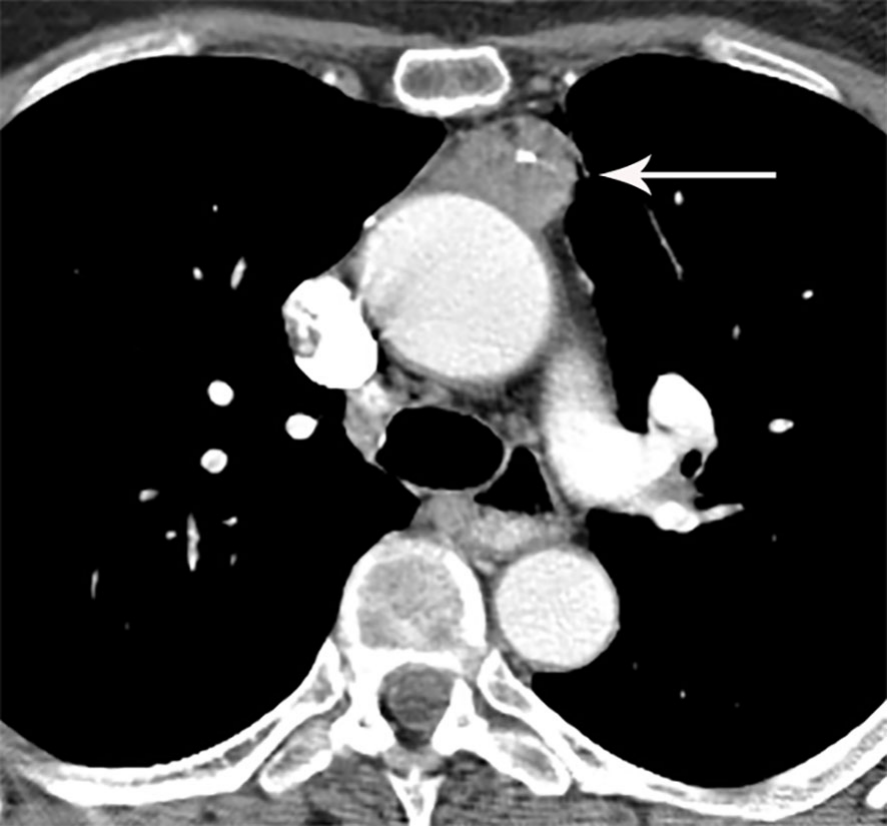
69 year old woman with thymic carcinoma. Contrast-enhanced CT shows left prevascular mediastinal mass (arrow) with small calcific focus.
